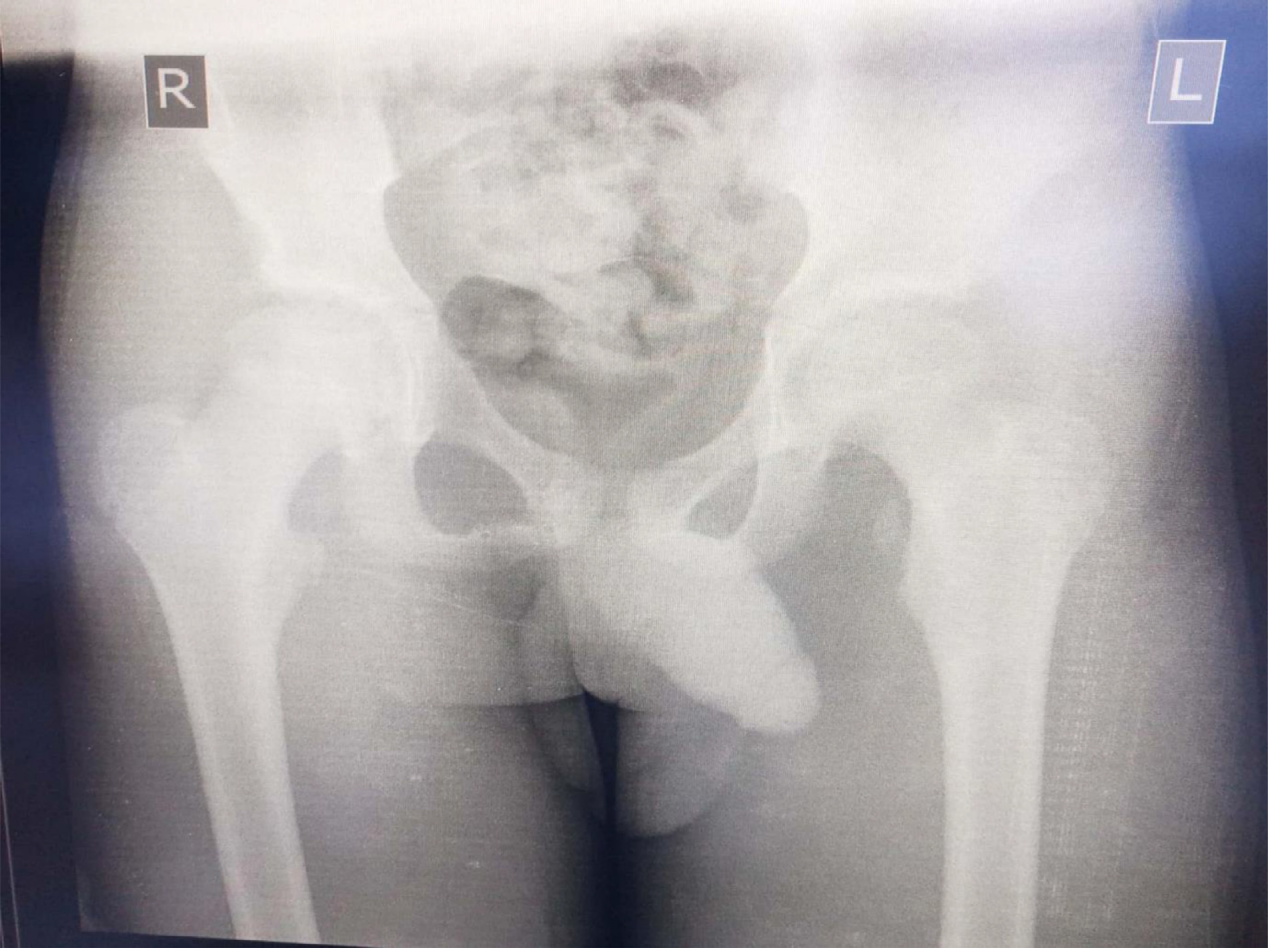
Radiograph of the pelvis of a 12-year-old patient showing an avulsion of the left lesser trochanter (arrow) compared to the right normal hip.
